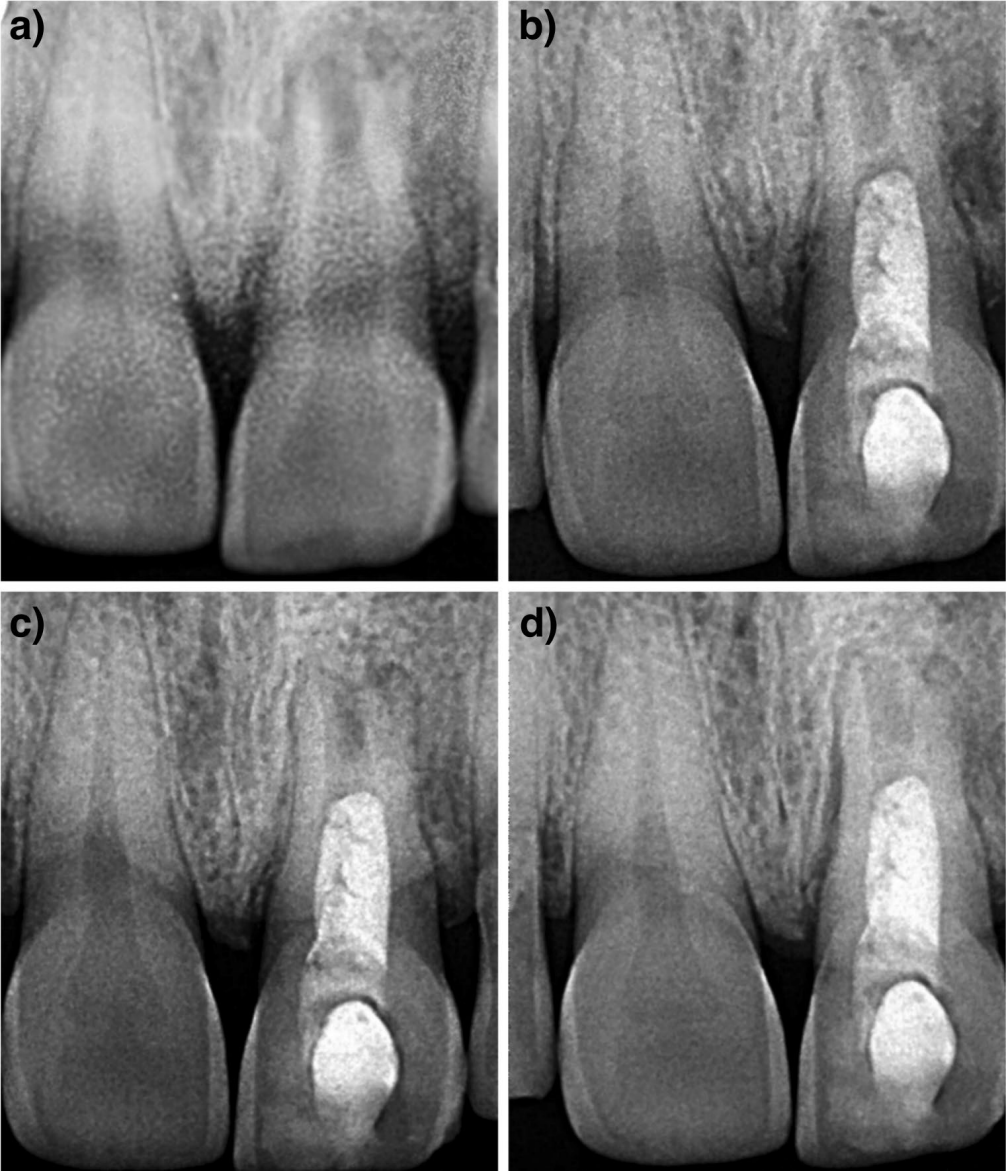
Case 3: Tooth no. 21- (A) Preoperative radiograph; (B–D) Radiographs at 6 months, 12 months and 24 months follow-up period.
